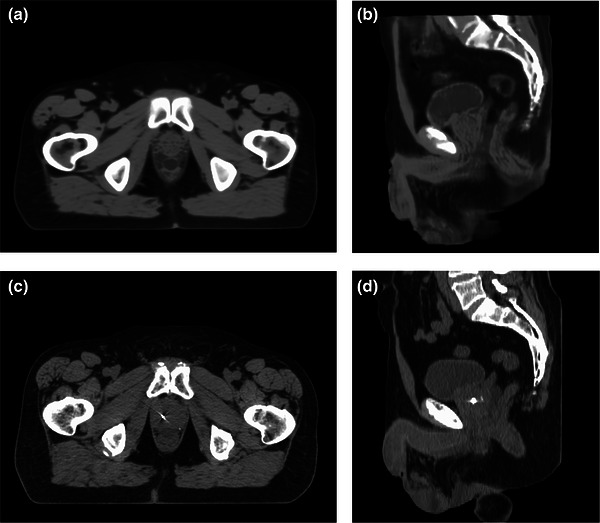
Prostate seed implants on the synthetic‐CT in (a) axial and (b) sagittal views. Implants on reference‐CT in (c) axial and (d) sagittal views. Seeds are absent in the sCT images.
